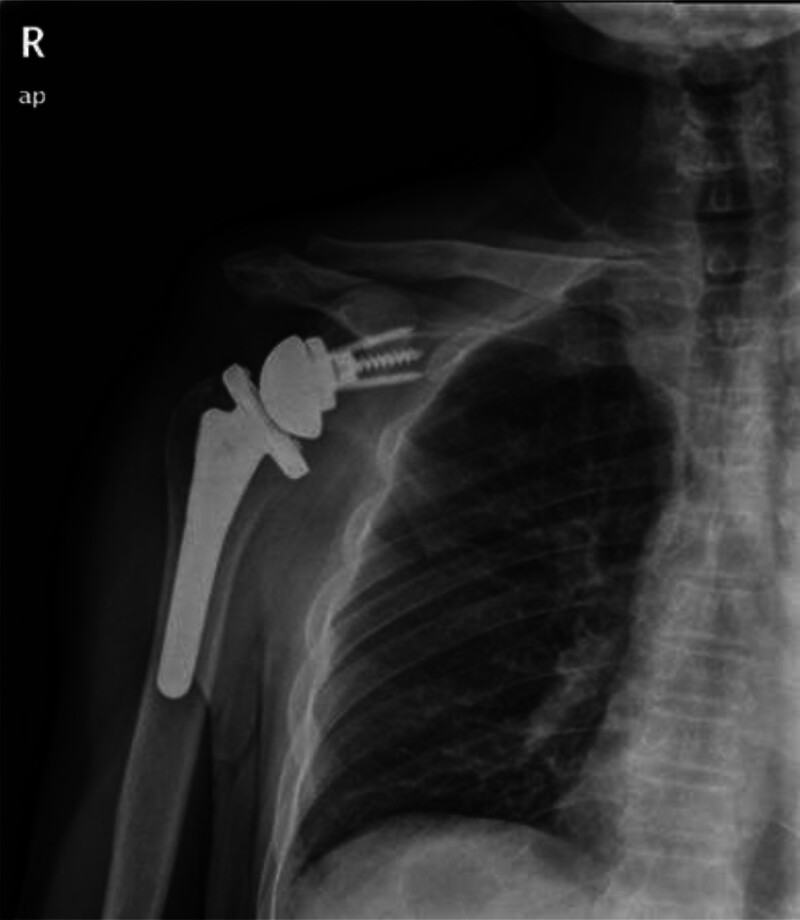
Postoperative X-ray follow-up at 1 year. The image shows the reverse total shoulder arthroplasty components (glenosphere, baseplate, humeral stem, and bearing) are well-positioned and appropriately fixed, with no radiographic signs of loosening, subsidence, or significant scapular notching.
